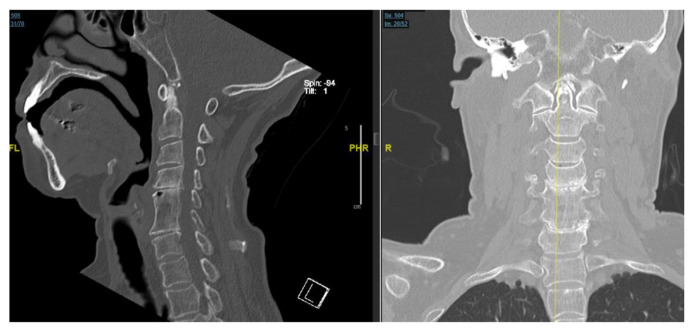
CT cervical spine demonstrating good bony fusion of C5–C6 and adjacent level degeneration from C3–C7.
